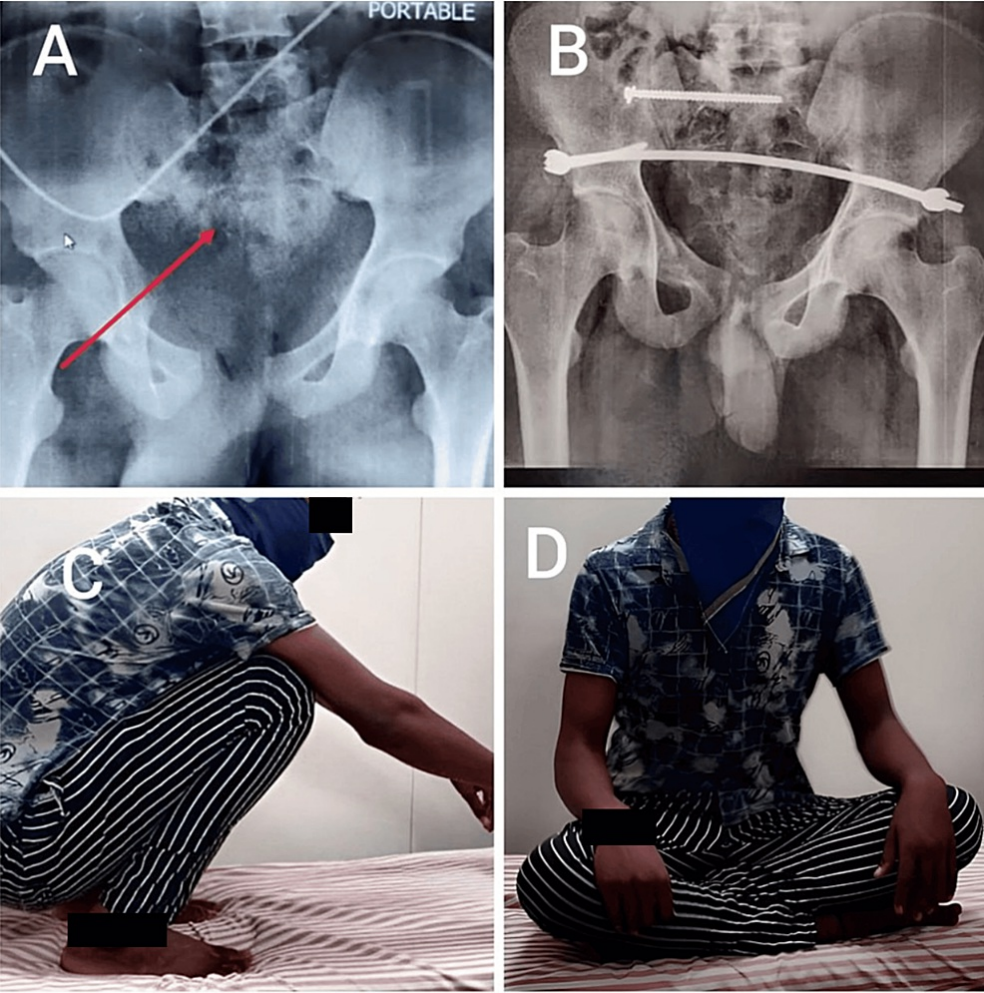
(A) Preoperative X-ray showing APC-II fracture; (B): postoperative X-ray of INFIX with SI joint screw; the patient squatting (C) and sitting cross-legged (D) six months after surgery INFIX: internal pelvic fixator; SI: sacroiliac
